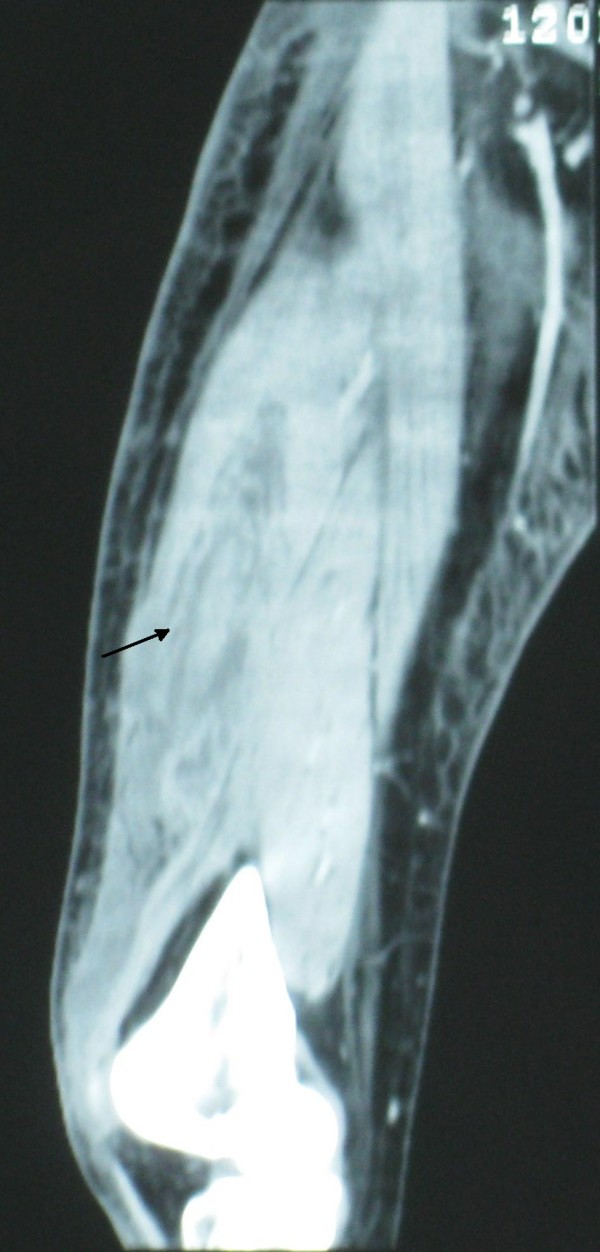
CT scan of right thigh, showing inflammatory stranding and low attenuation in vastus latralis (arrow).
